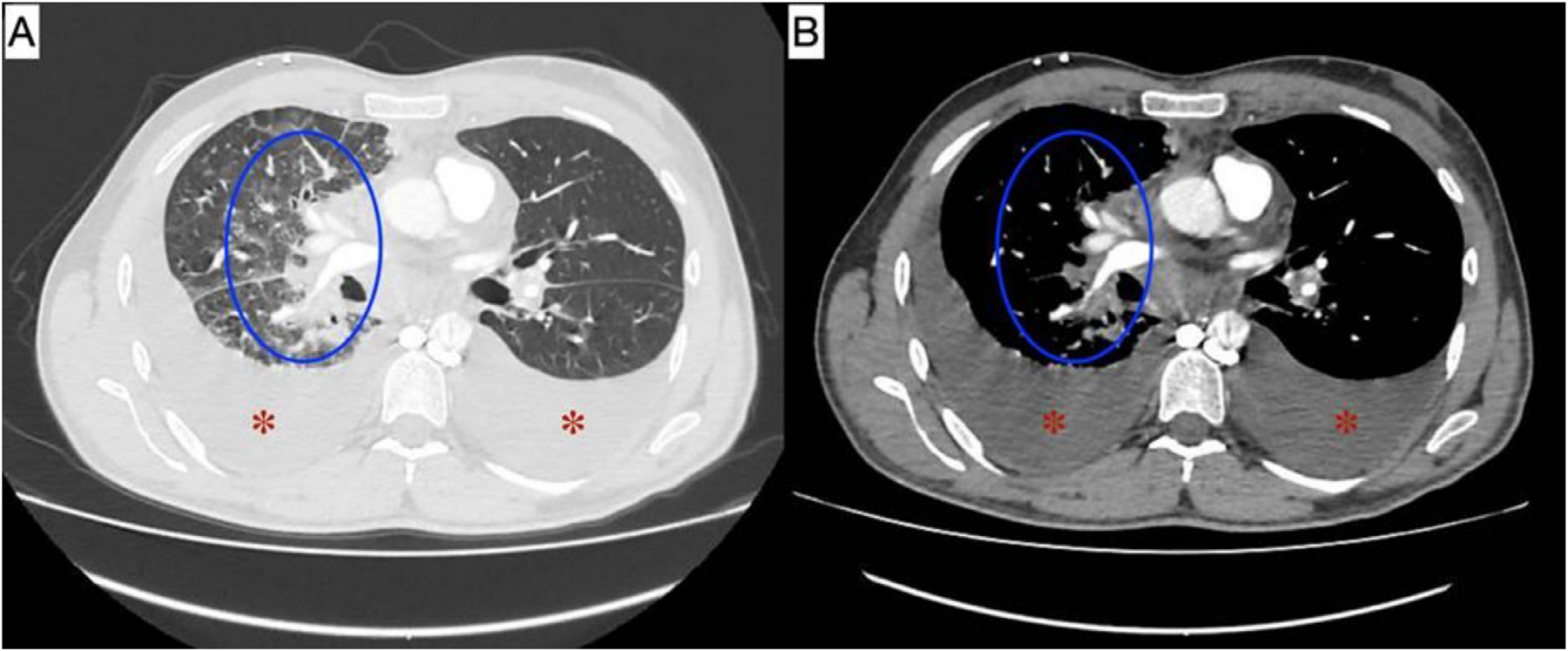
(A) Lung window CT; (B) Mediastinal window CT. Blue circles represent areas of perihilar consolidation accompanied by surrounding ground-glass opacities and architectural distortion, which are characteristic radiological features of lung adenocarcinoma. Burgundy asterisks denote the presence of bilateral pleural effusion in both windows.
